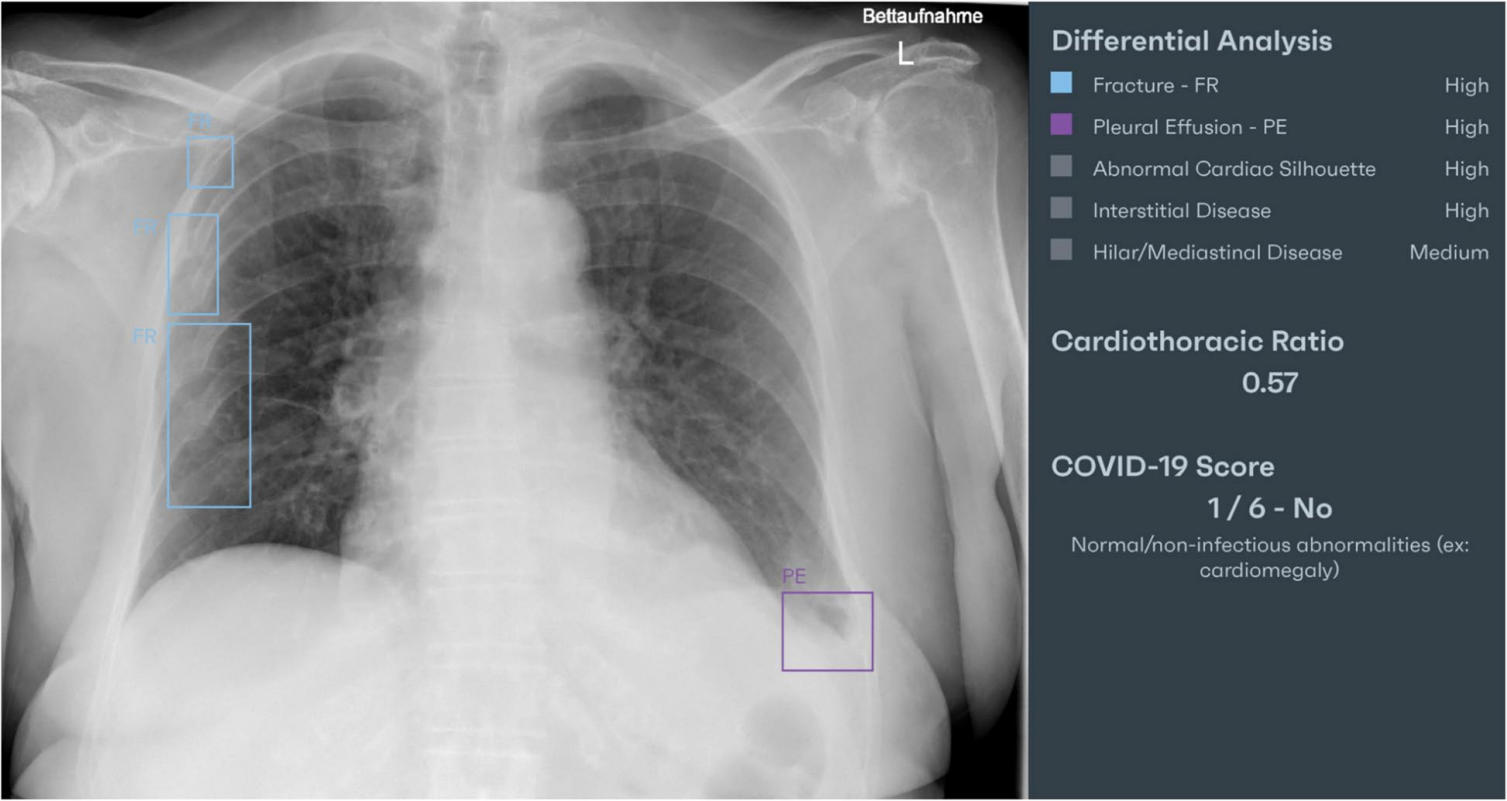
Chest X-ray with marked AI findings
